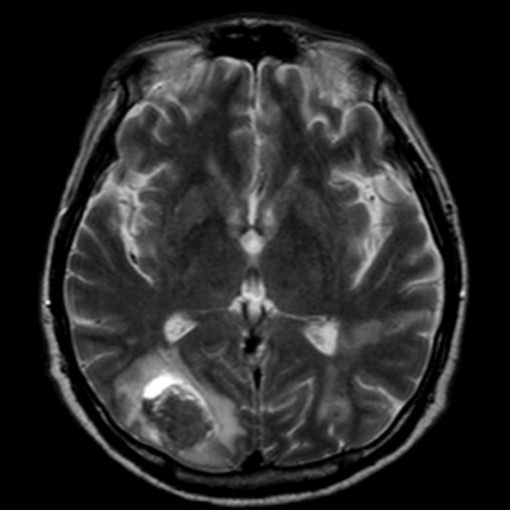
Post-operative MRI showed a large cerebral haemorrhagic infarction in the right occipital lobe.
